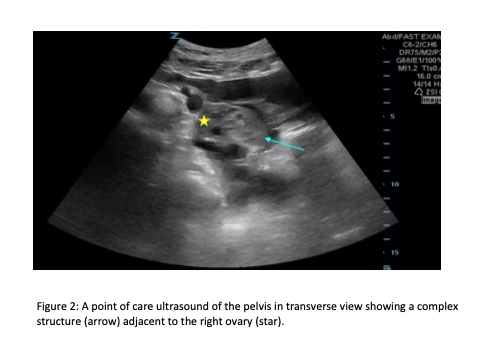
Point-of-care ultrasound of the pelvis in transverse view showing a complex structure (arrow) adjacent to the right ovary (star).
